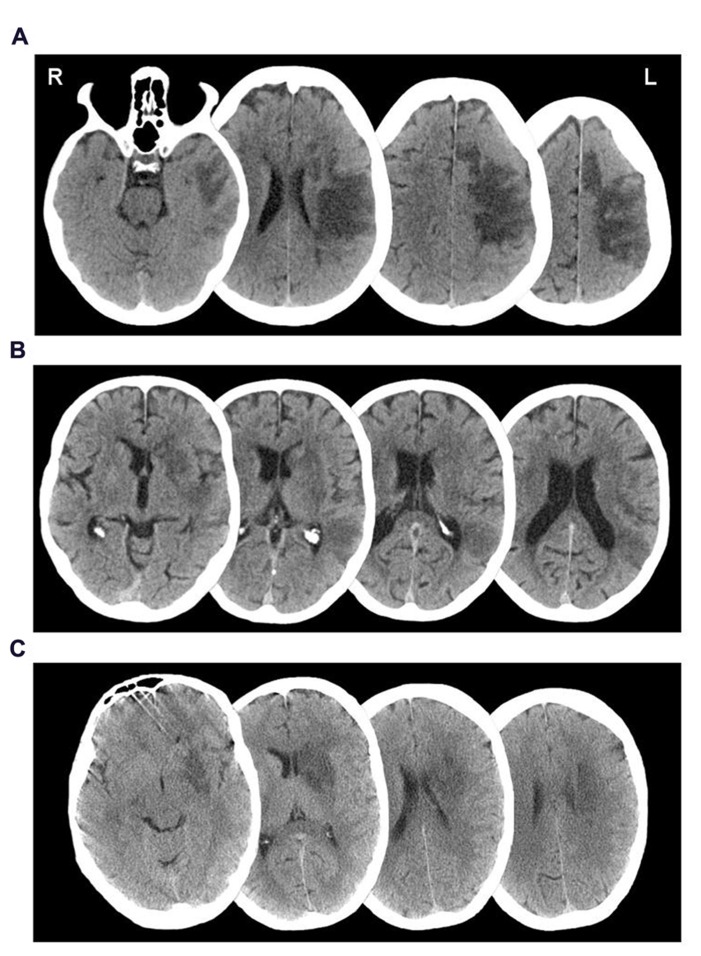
Axial CT imageshowing lesion distributions of participants (A) 001,(B) 002, and (C) 003.
